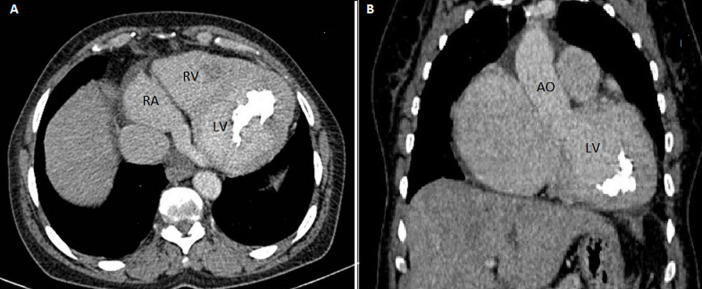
computed tomography (CT) cardiac scan; A) 4-chambre view; B) two chambre view shows a left ventricle apical obliteration due to a massive calcification which reached the posterior-medial papillary muscle
